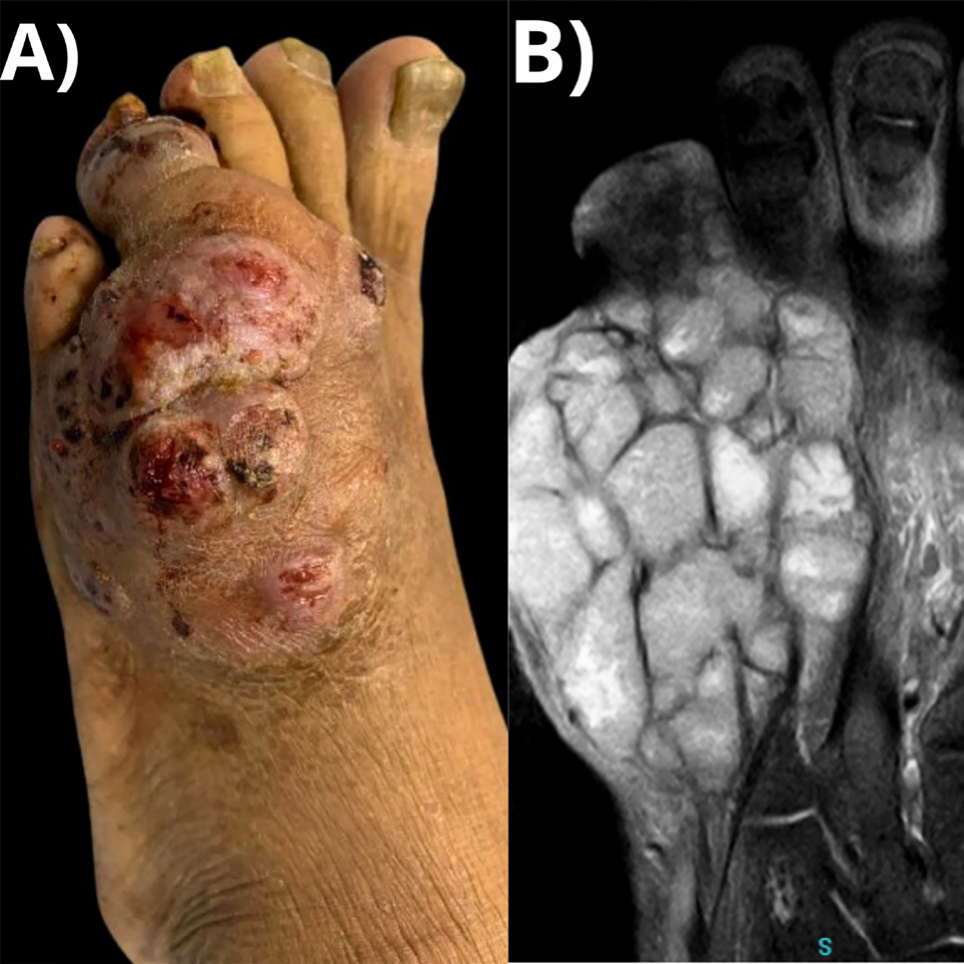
A) Tumor with lobulated surface and fistulous ostia, with drainage of serosanguineous material, without grains, located on the dorsum of the left foot; B) Magnetic resonance imaging depicting multiple septa and nodules affecting deep tissue planes, containing exudate, without bone involvement.
